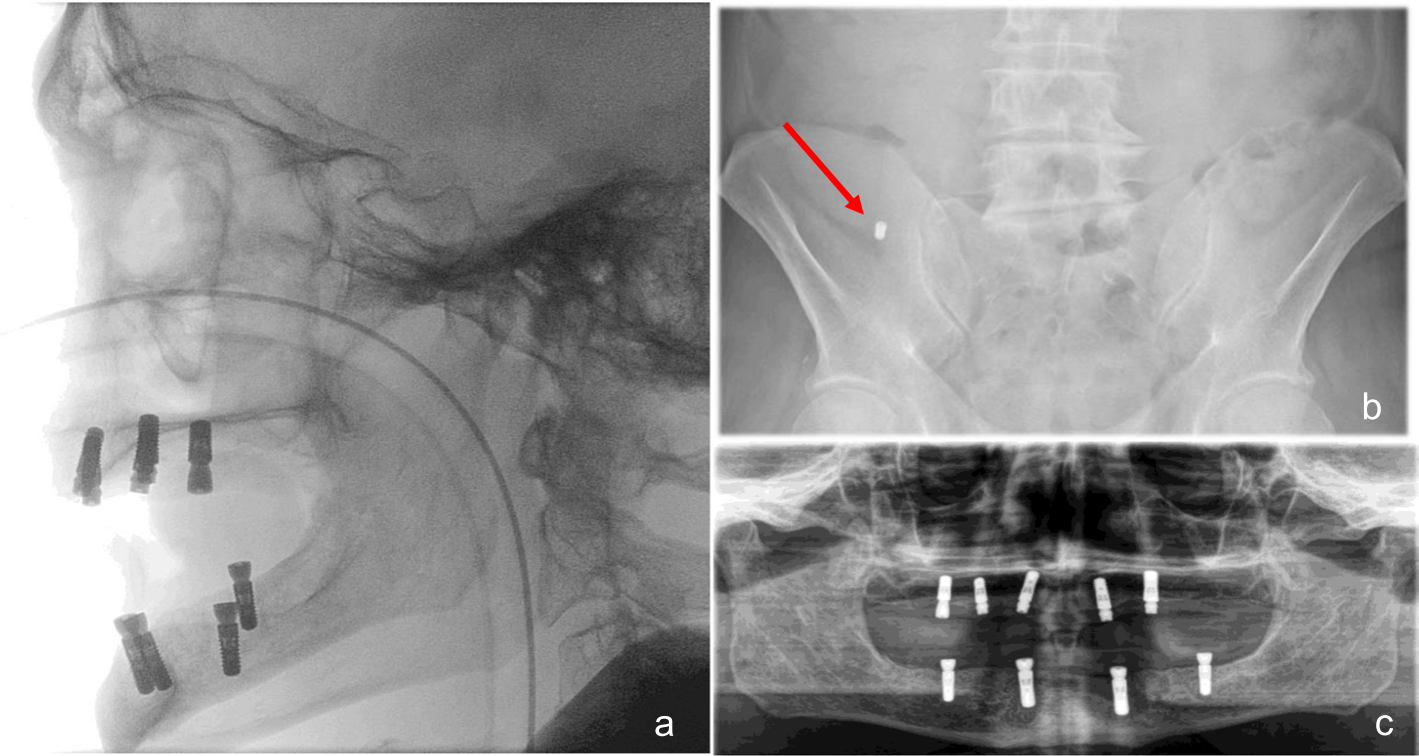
Case 3 – postoperative imaging for detection of the migrated implant. a Intraoperative C-arm X-ray. b Postoperative Abdominal X-ray: Revealed the displaced implant in the ascending colon, suggesting spontaneous expulsion and ingestion. c Postoperative Panoramic radiograph: Displays the osseointegrated implants and the bony defect in the left upper second molar region
